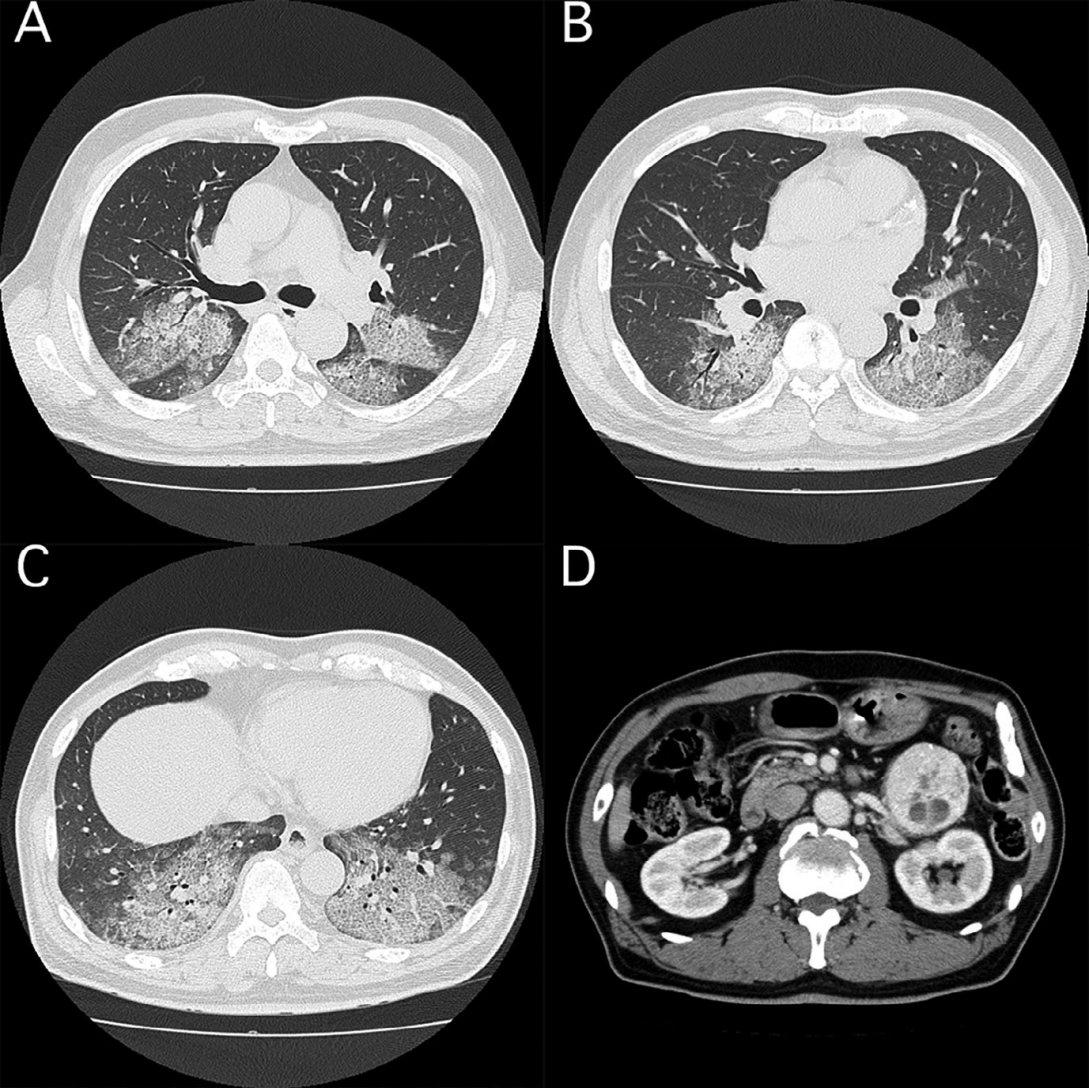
Thoracic‐abdominal computed tomography (CT) findings. CT scan showing crazy‐paving appearance (ground‐glass opacities and interlobular septal thickening) in bilateral lungs (A–C) and heterogeneous left adrenal lesion (D).
